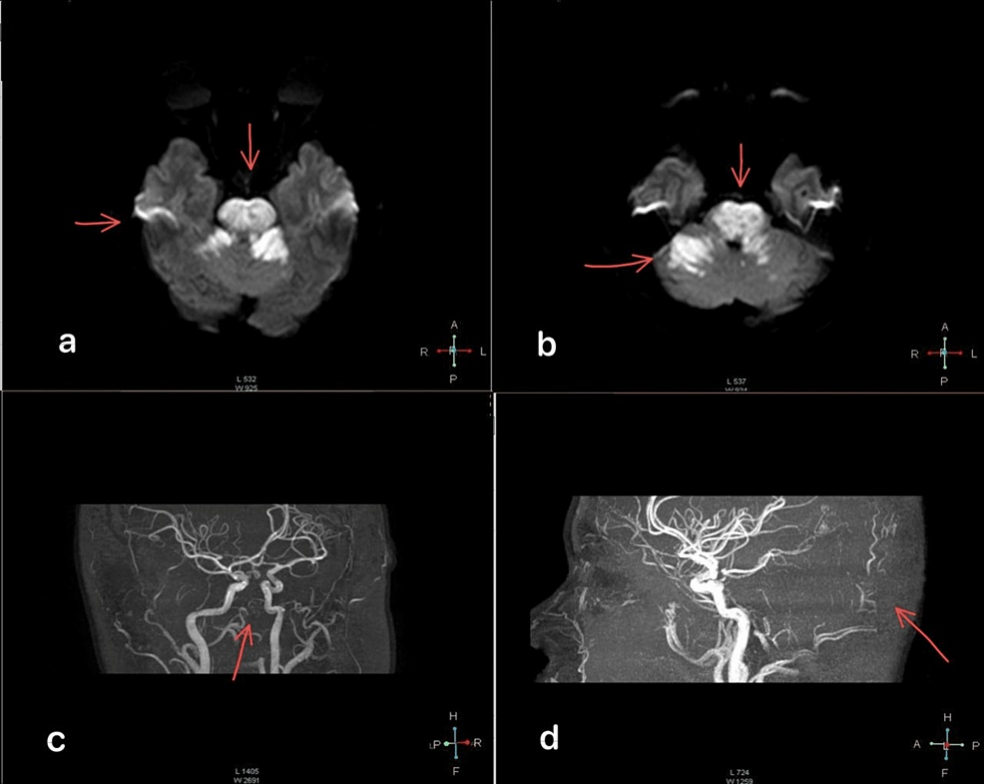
Magnetic resonance image (MRI) in DWI and magnetic resonance angiographic (a) DWI (diffusion-weighted imaging) MRI showing extensive restricted areas in the pons and cerebellar hemispheres. (b) DWI MRI in lower sections continuing to display these restricted areas. (c, d) Magnetic resonance angiographic (MRA) reveals the absence of vascular pathways in the posterior circulation, with no visualization of flow in the basilar artery or its branches.
